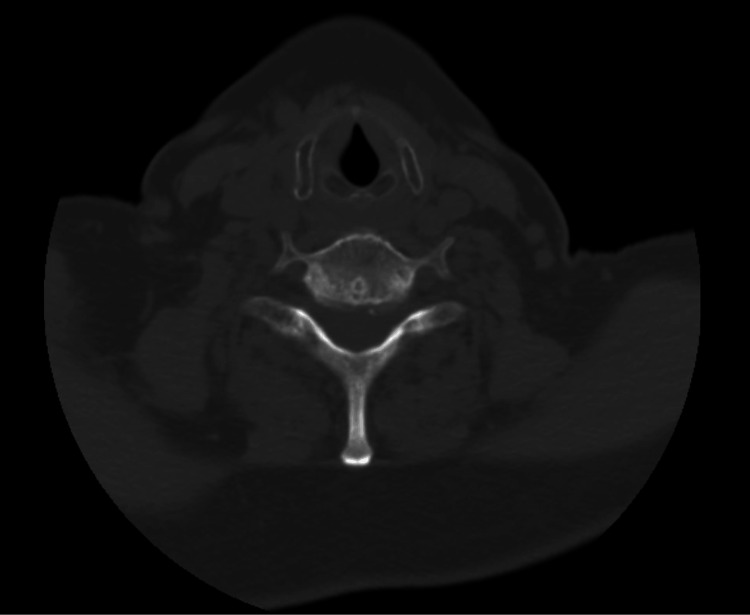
Axial non-contrast CT scan at the C5–C6 level demonstrating normal cervical spine anatomy. The vertebral bodies, spinal canal, and surrounding soft tissues appear unremarkable, with no evidence of fracture, malalignment, canal stenosis, or acute soft-tissue abnormality.
